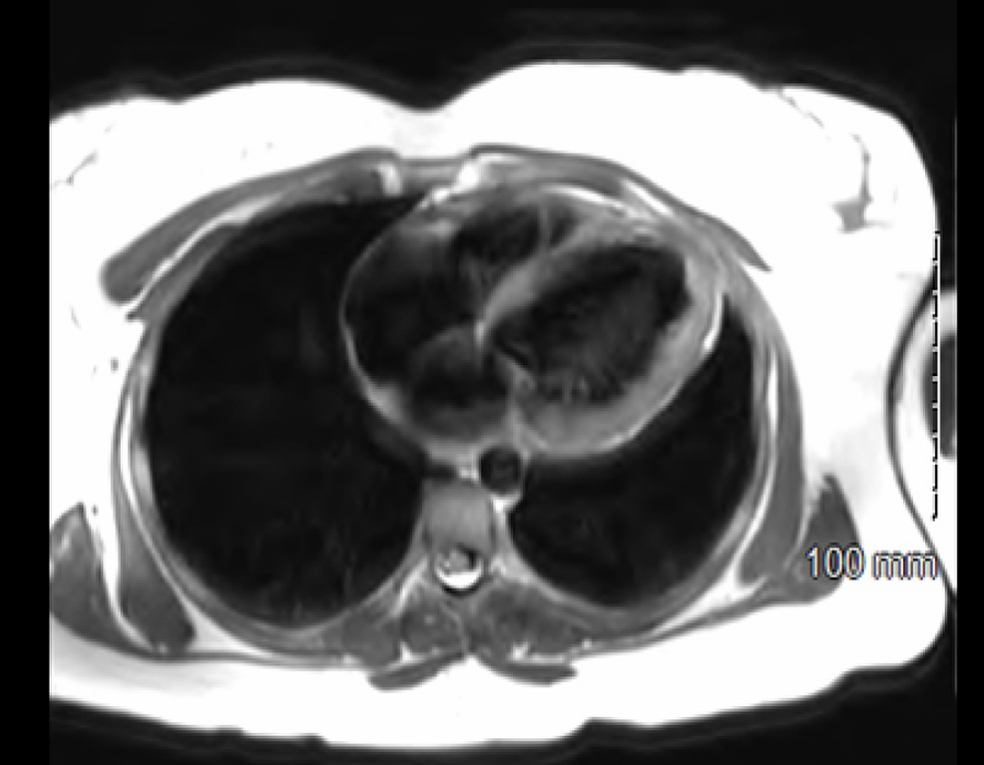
Cardiac MRI Cardiac MRI showed findings of epicardial enhancement and edema compatible with apical anterior lateral wall myocarditis
